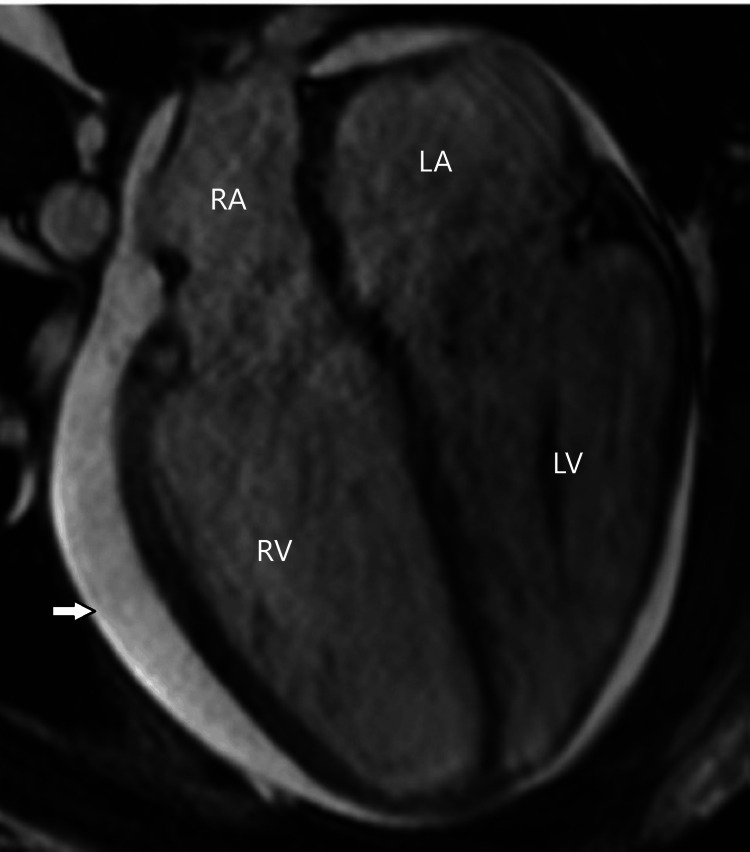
Cardiac Magnetic Resonance Imaging (MRI). Still frame of a four-chamber steady-state free precession (SSFP) cine MRI showing normal left ventricular size, mild right ventricular dilation, and small to moderate size pericardial effusion (arrow). RA: right atrium; RV: right ventricle; LA: left atrium; LV: left ventricle
